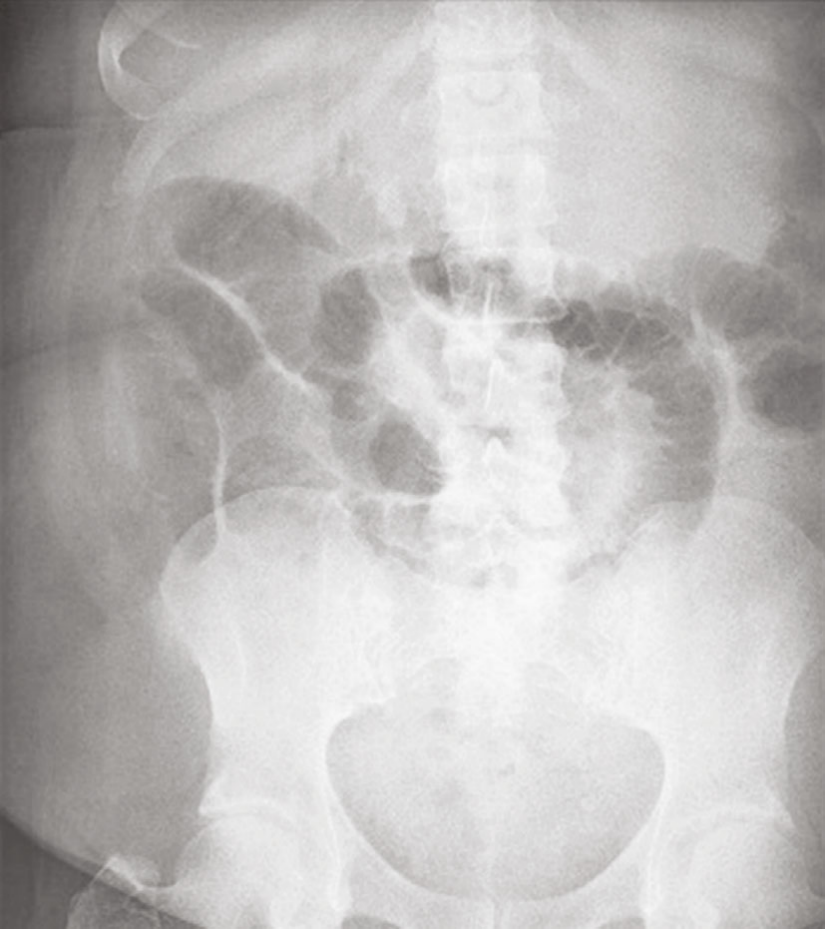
AP supine X-ray of the abdomen showing moderate gaseous distention of the small bowel.
